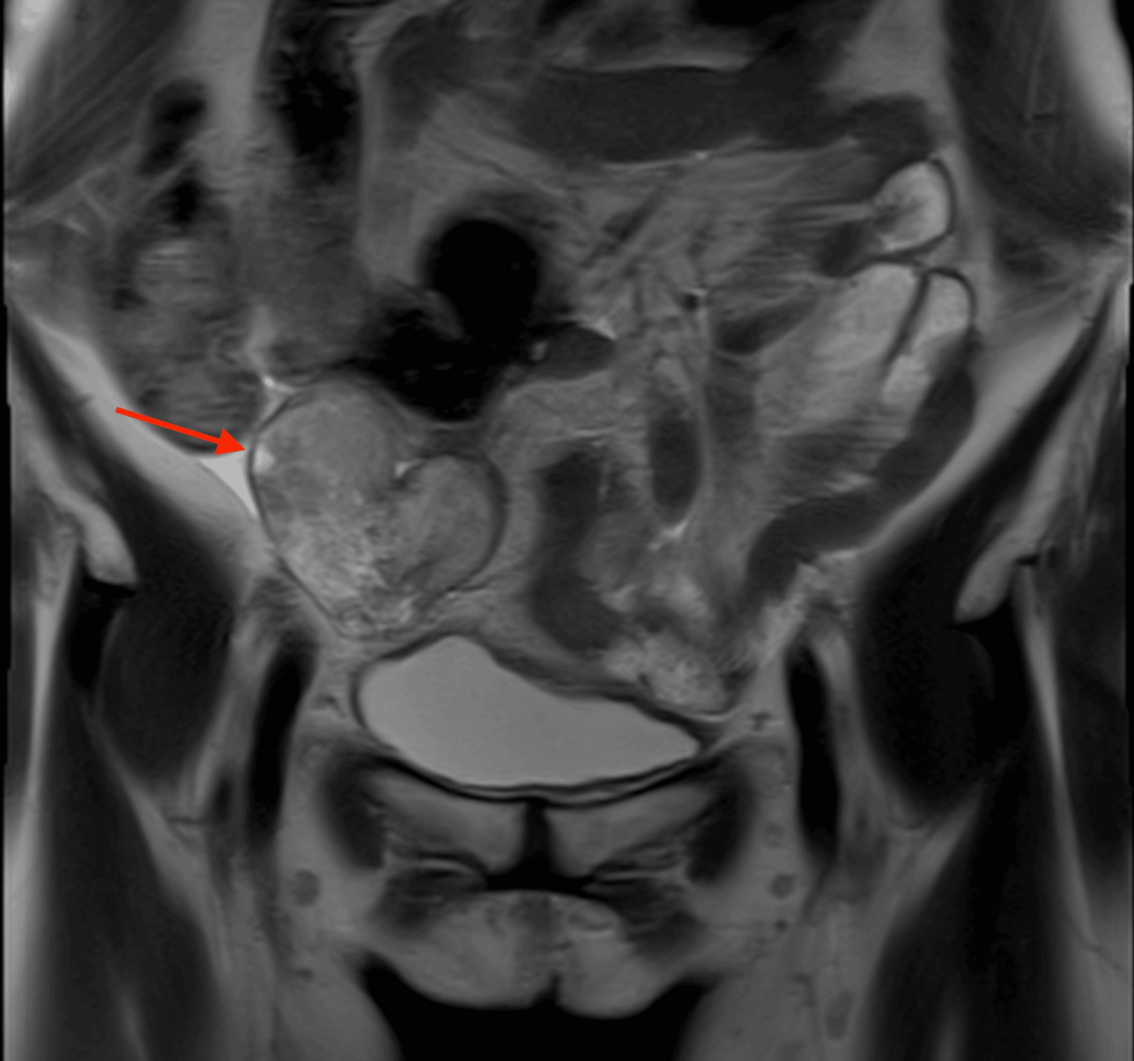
MRI in a 62-year-old woman with ovarian carcinosarcoma of the right ovary A heart-shaped tumor with well-defined margins and predominantly solid components is observed on the coronal T2-weighted image (arrow). The tumor measures 5.7 cm in diameter.
